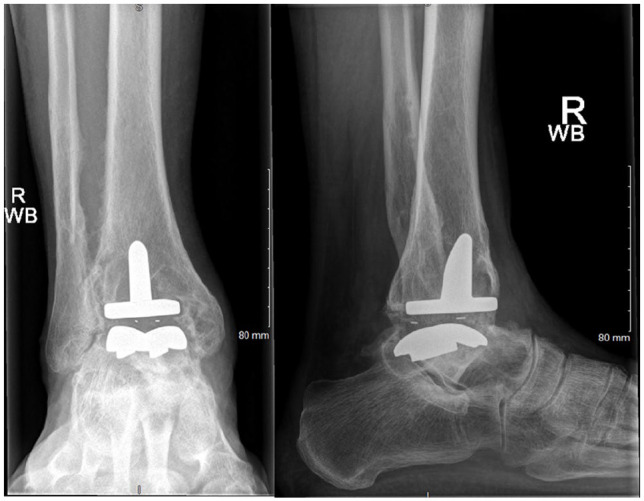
Preoperative radiographs of failed total ankle arthroplasty (Ankle Evolutive System) with large bony defect measuring >4 cm.
